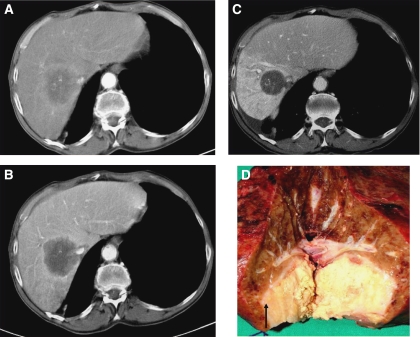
Contrast-enhanced pre-radioembolisation arterial (A) and portal-venous-phase (B) CT scans showing large CRC liver metastasis. 6-month post-radioembolisation (C) including significant attenuation, sharp margins and thin peripheral enhancement compatible with complete lesion necrosis; confirmed by post-resection evaluation (D), showing fibrotic capsule (arrow).
